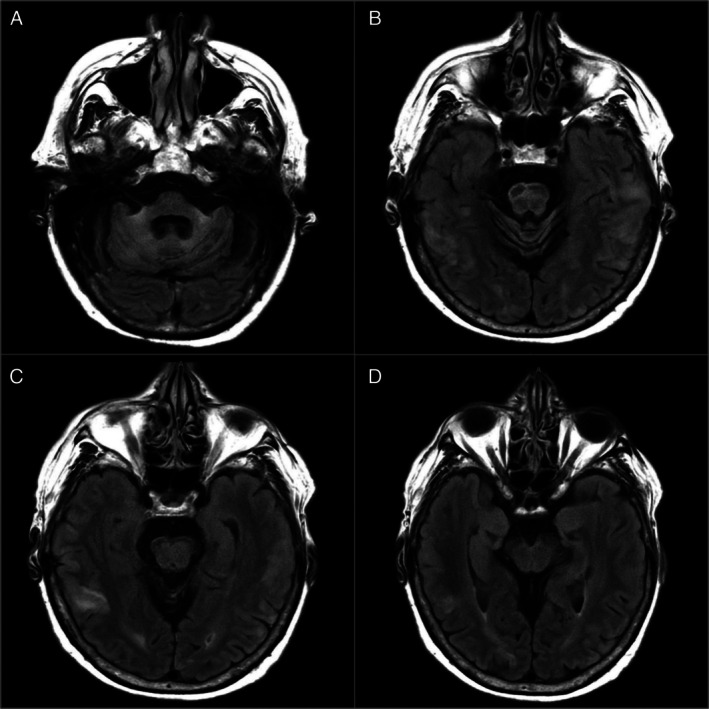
T2/FLAIR images obtained on presentation to the emergency department with acute hypertension and confusion. Note significant gliosis of the middle cerebellar peduncle (A) and prominent pontine cruciform hyperintensity or “hot cross buns sign” (B). Additionally, there are several bilateral temporal and occipital lesions (B–D), predominantly posterior, highly suggestive of posterior reversible encephalopathy syndrome (PRES), most prominent in the R temporal lobe (C).
